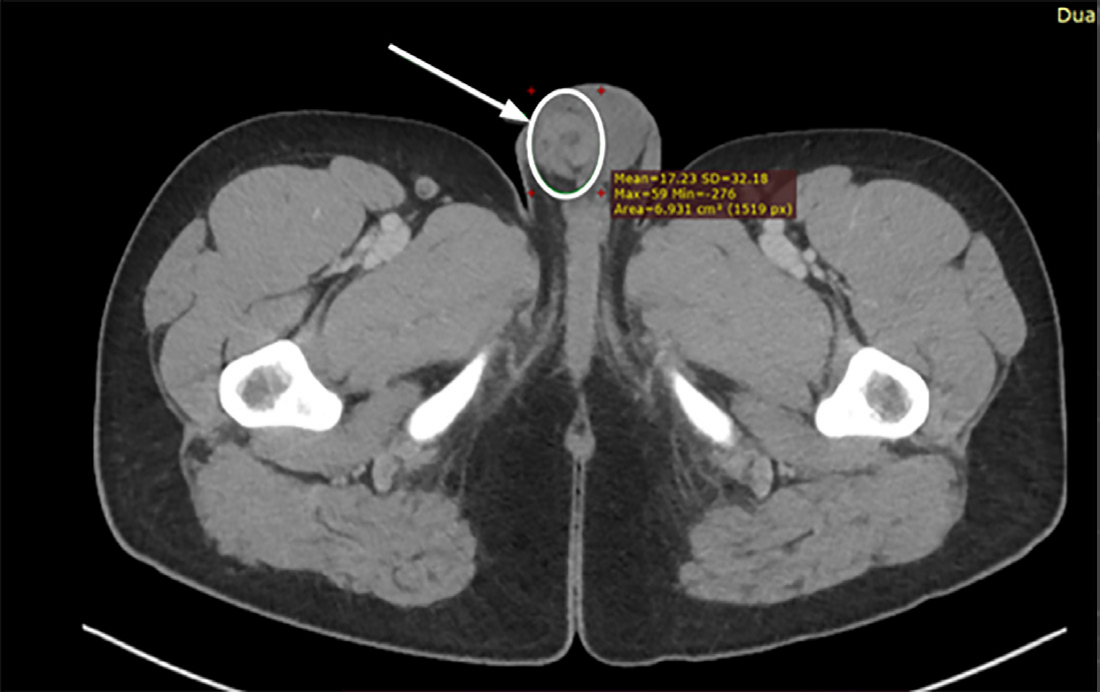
Axial computed tomography of the abdomen and pelvis showing “whirl” sign in the right scrotum at the inferior periphery of the image suggestive of testicular torsion. The white arrow and circle highlight this finding.
